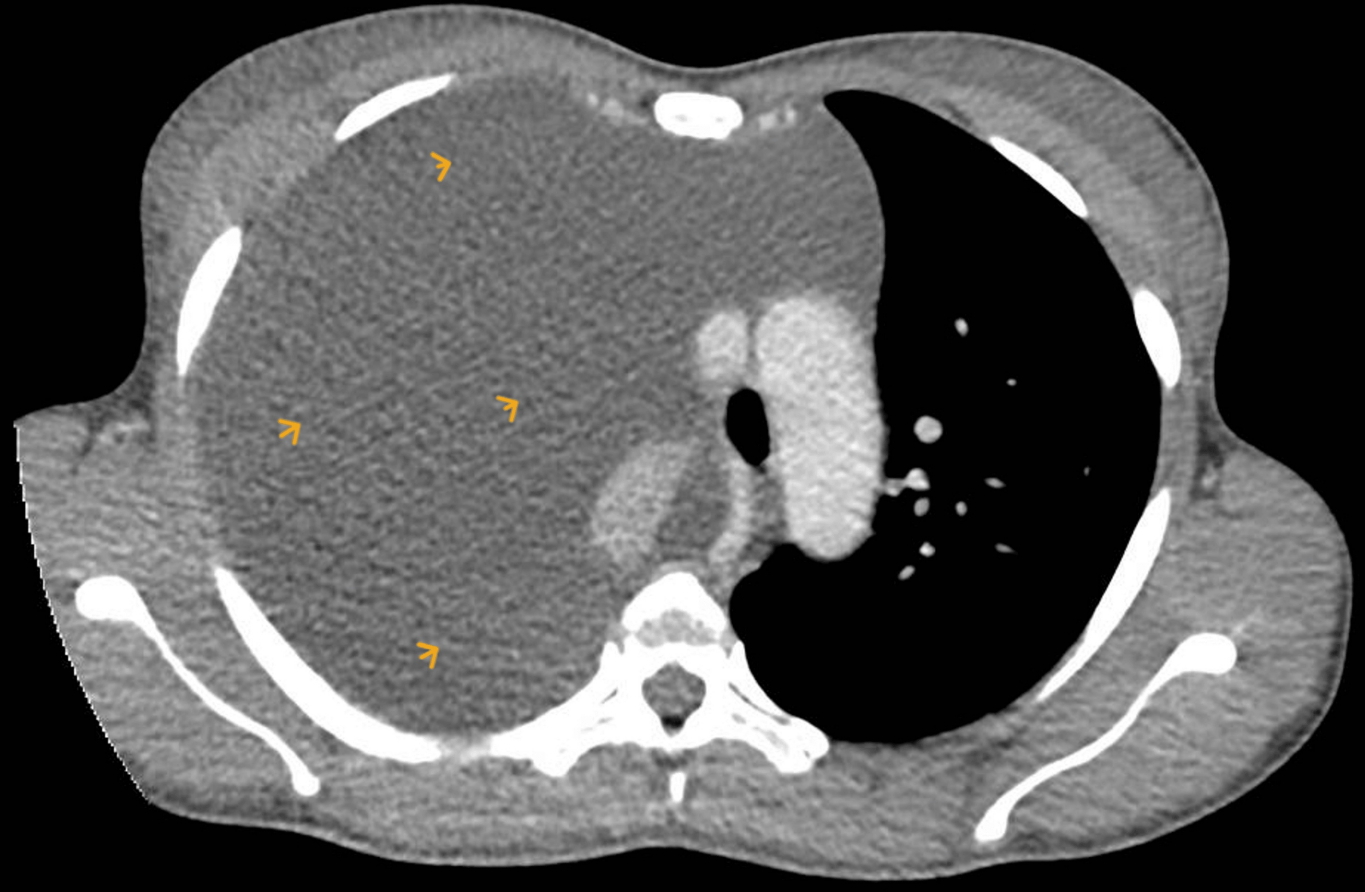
CT scan showing a large volume right-sided pleural effusion (yellow arrows) CT: computed tomography
